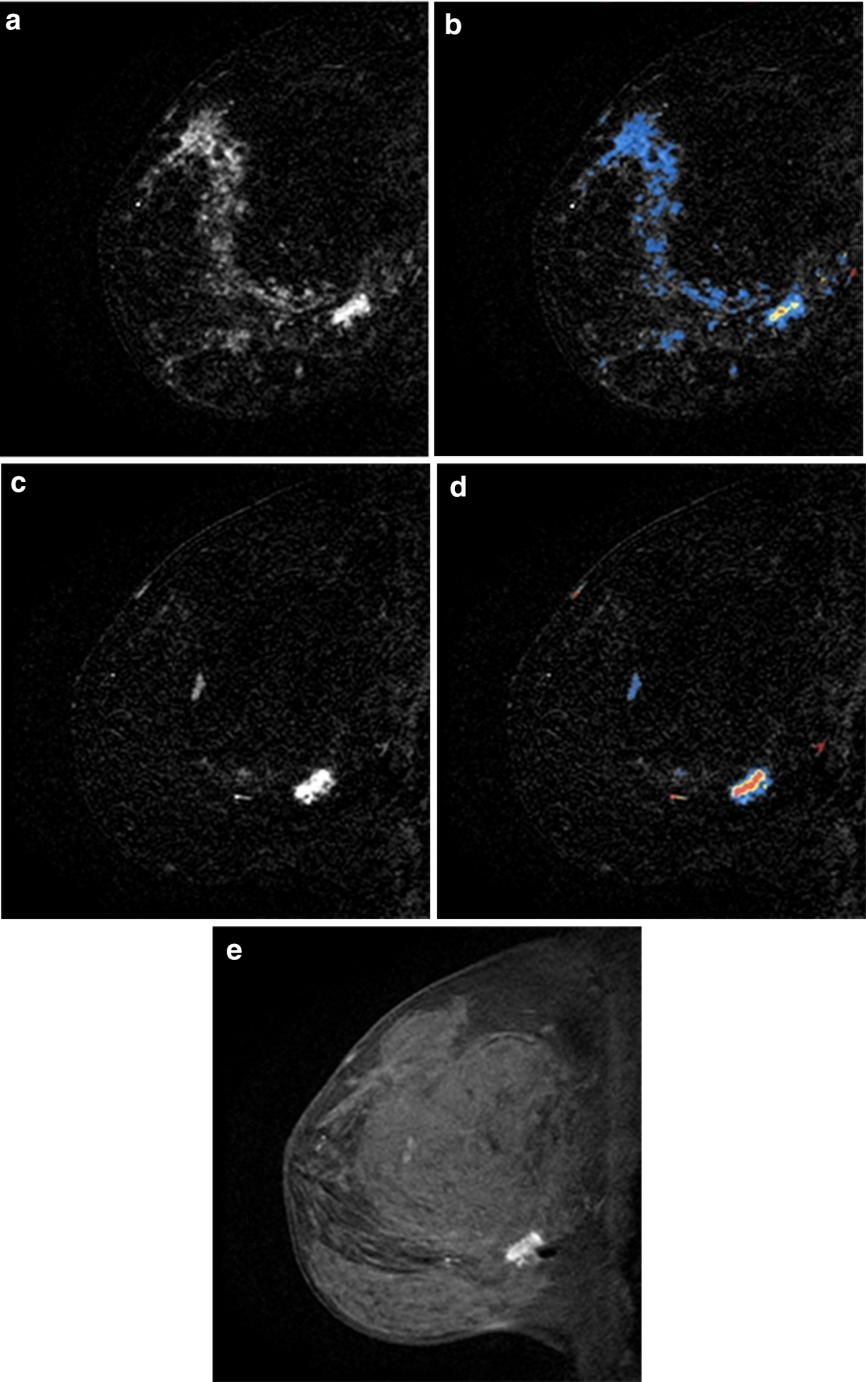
Missed MRI-guided biopsy with follow-up demonstrating cancer. a Postcontrast subtraction T1-weighted image shows a 1.2-cm non-mass enhancement (NME) with focal distribution, heterogeneous internal enhancement, and b washout kinetics (arrow), which was suspicious and assessed as BI-RADS 4. MRI-guided biopsy was performed yielding fibrocystic changes and a 6-month follow-up MRI was recommended. At 6-month follow-up, c postcontrast subtraction T1-weighted image shows persistence of the NME and washout kinetics (d). Postcontrast T1-weighted image (e) shows that the susceptibility artifact from the biopsy marker clip is located posterior to the focal NME, which was unchanged in size and appearance suggesting that the NME was not biopsied. Surgical excision yielded carcinoma in situ
